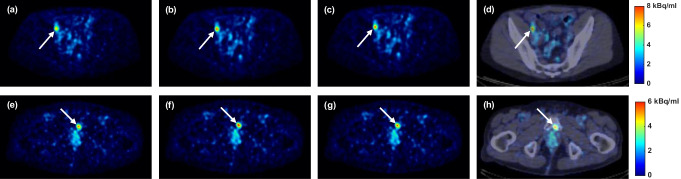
Image comparison of soft tissue and bone lesions. From left to right, the columns show non-PRC, tissue-independent PRC, TDSV PRC, and PET/CT fusion for the TDSV PRC. Soft tissue lesion (lymph node): (a)-(d). Bone lesion (metastasis): (e)-(h)
